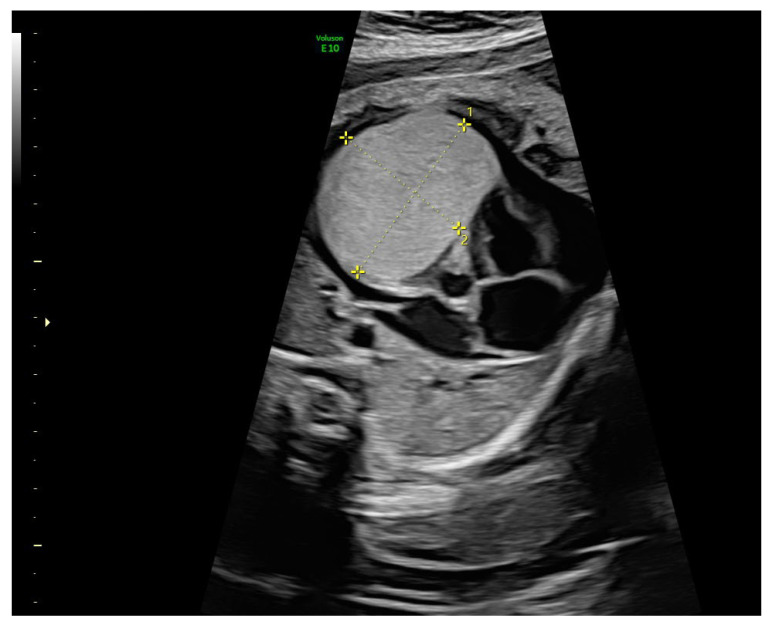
Axial transthoracic view showing a hyperechoic cardiac mass (31.98 × 23.97 × 36.78 mm) with well-defined borders and a mess effect consistent with a cardiac rhabdomyoma. Cardiomegaly and pericardial effusion are also observed.
